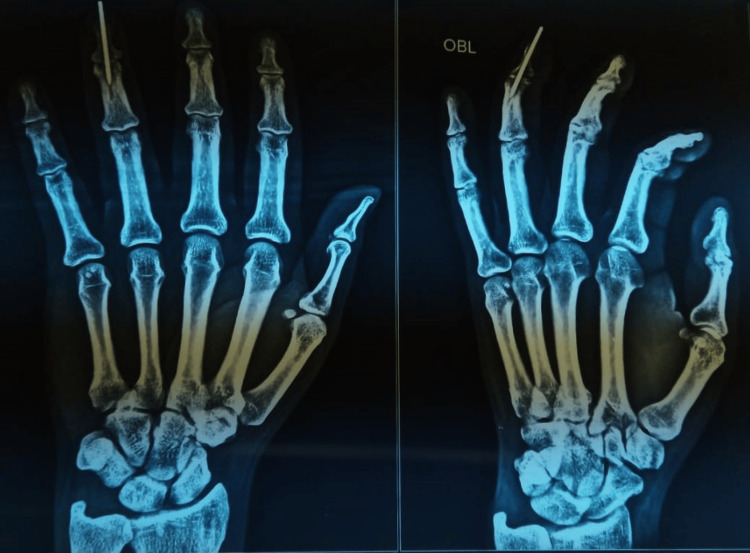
Postoperative radiograph showing AP and lateral views
